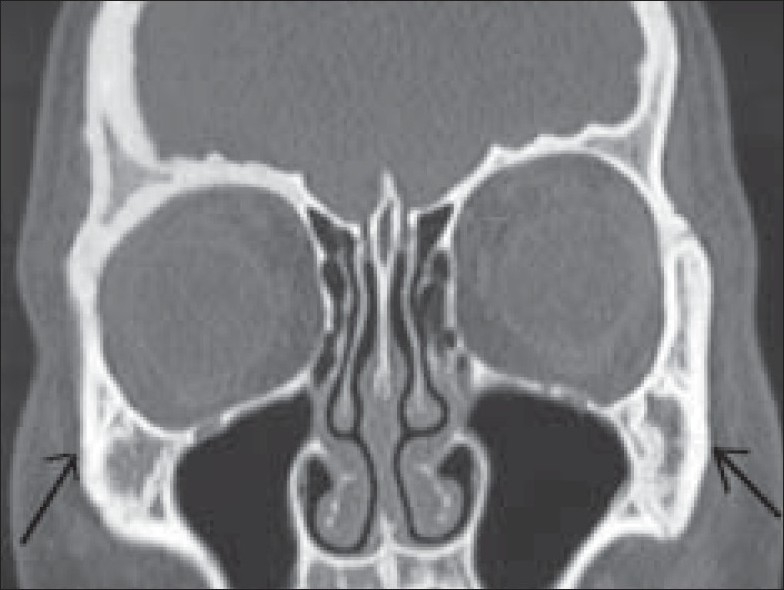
Patient 2 – CT scan of the orbit showing osteolytic lesion in both lateral orbital wall
